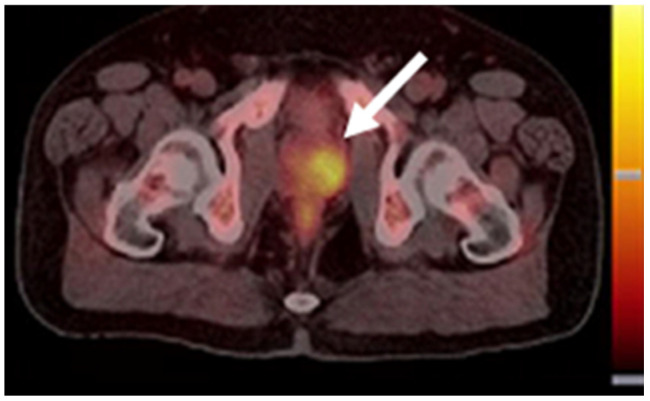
[^11^C]Choline PET/CT image of a patient with an adenocarcinoma of the prostate (white arrow). This research was originally published in the Journal of Nuclear Medicine (JNP) [29].
